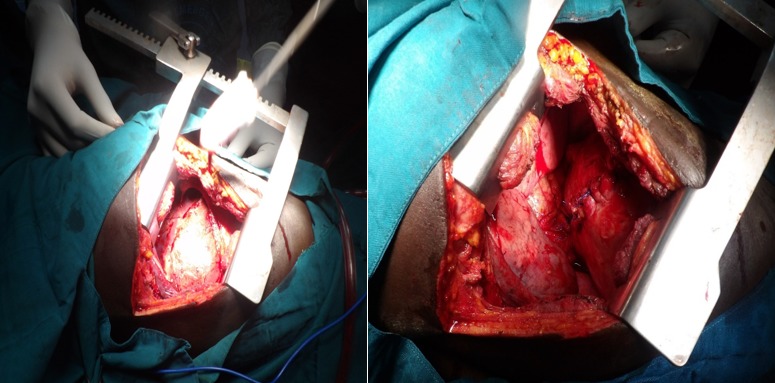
Postoperative erect chest x-ray of case 2 showing adequate bilateral lung expansion
